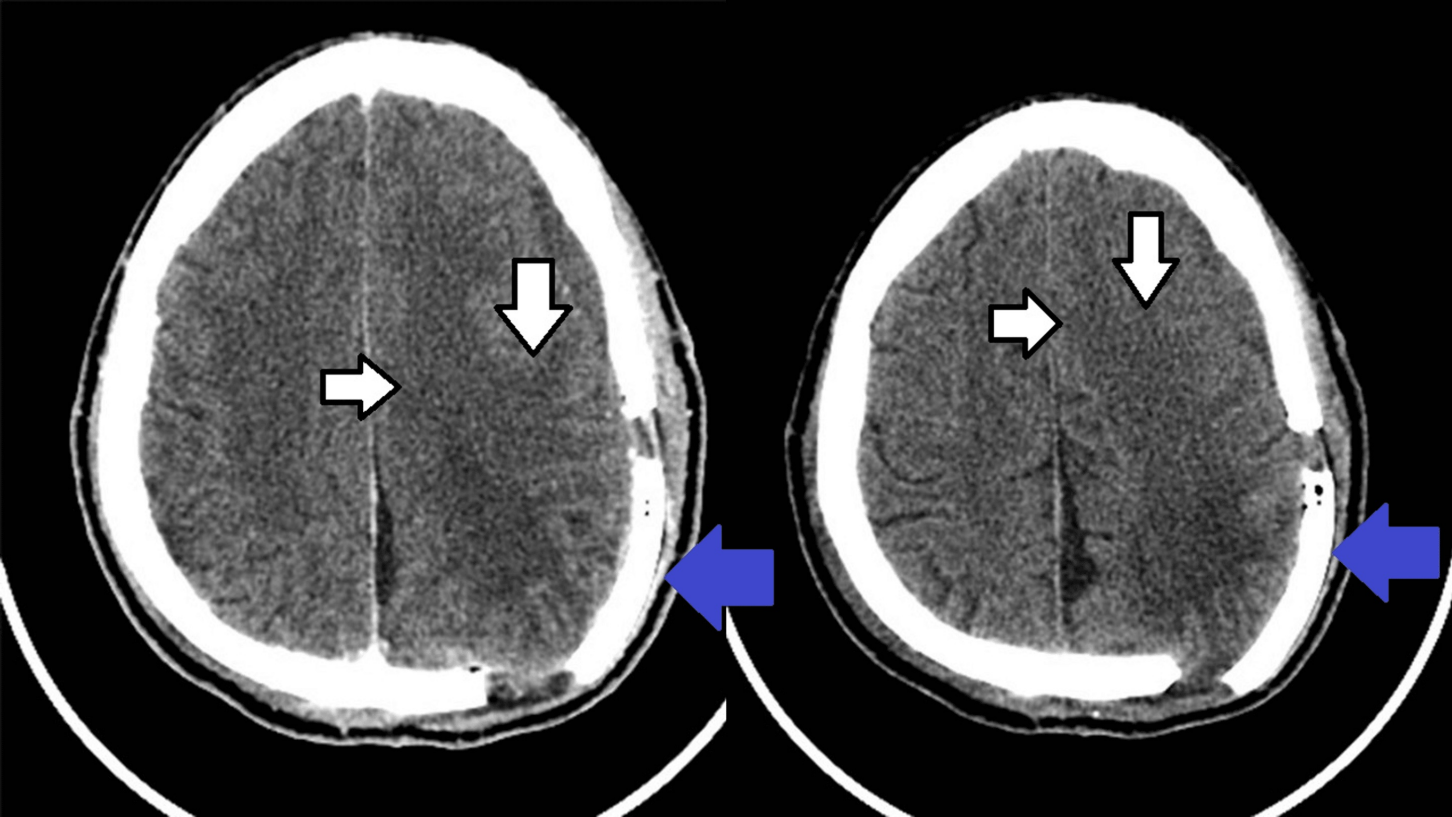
Non-contrast head CT scan showing the site of osteoplastic craniotomy (blue arrows) with gross total resection of the lesion and postoperative edema (white arrows with black outline).
